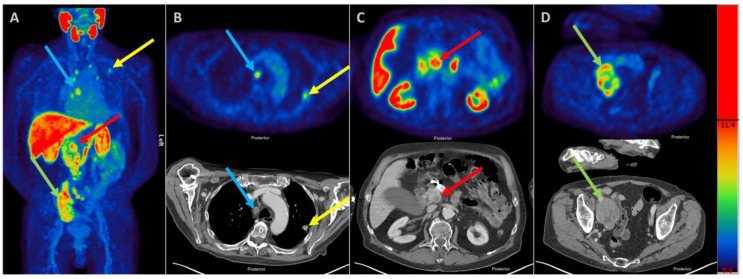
18F-PSMA PET/CT scan of a patient with known prostate cancer (maximum intensity projection (A)). Multilocular PSMA positive tumor lesions were revealed in the lung (B), the mediastinal (B), the pancreas (C) as well as in abdominal/iliac lymph nodes (D).
